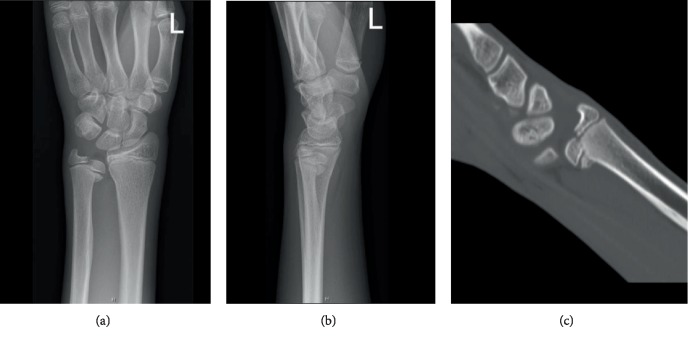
(a, b) Anteroposterior and lateral X-ray after trauma showing an isolated Salter-Harris type IV fracture of the ulna. (c) Sagittal CT image showing an intraarticular step of 3 mm.
